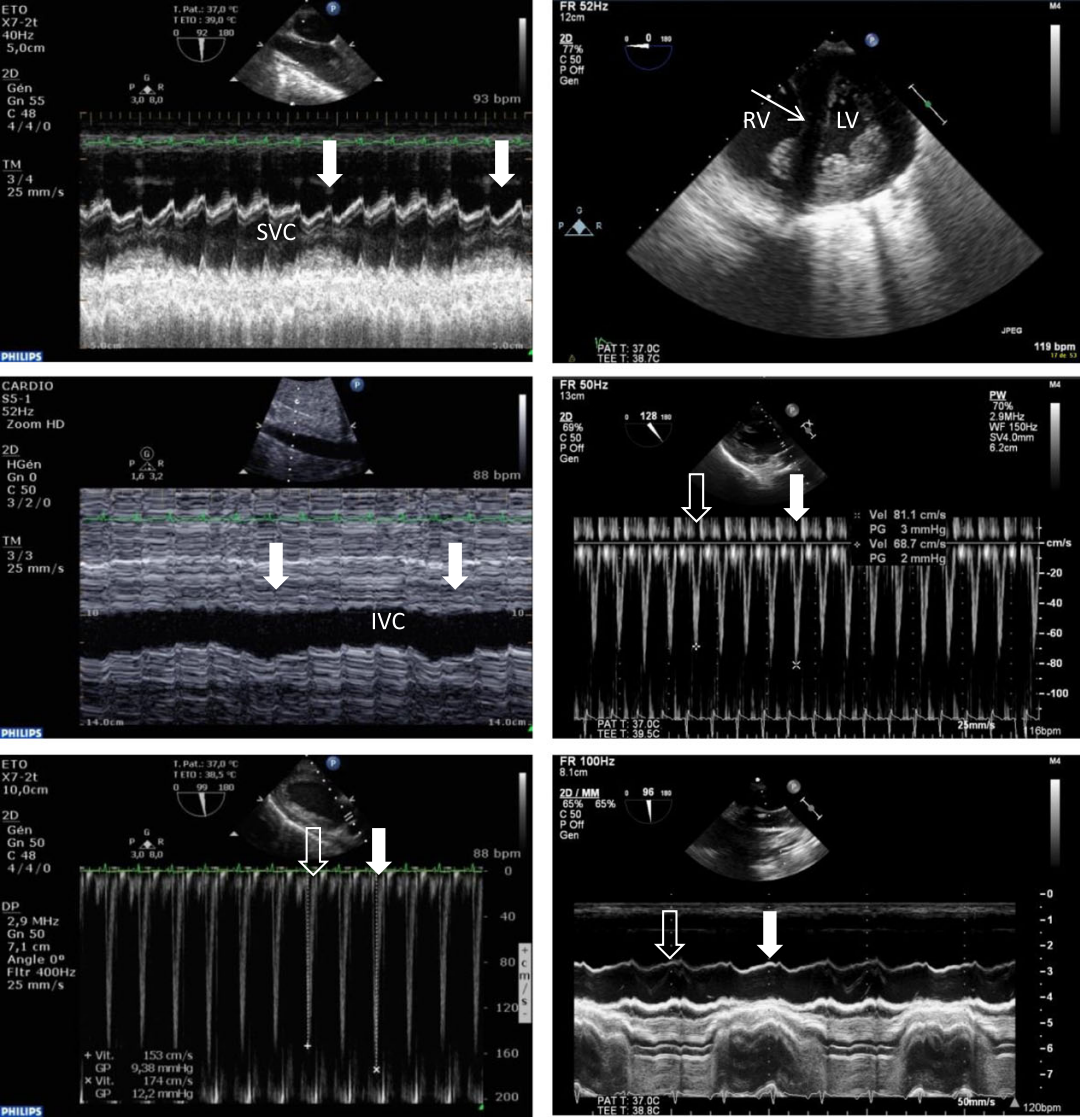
Hemodynamic assessment using transesophageal echocardiography in two patients presenting with shock of distinct origin. In the first patient (left panels), inadequate cardiac output was ascribed to a persisting preload dependence, as reflected by concordant and significant respiratory variations (closed thick white arrow: mechanical insufflation) of the superior vena cava (SVC) diameter (upper left, collapsibility index), of the inferior vena cava (IVC; middle left, distensibility index), and of maximal Doppler velocity recorded in the left ventricular outflow tract (lower left, open thick arrow: expiration phase). In this patient, cardiac output significantly increased after fluid loading. In the second patient who was under protective mechanical ventilation for an acute respiratory distress syndrome (right panels) and exhibited tissue hypoperfusion, a 15% pulse pressure variation suggested fluid responsiveness. Transesophageal echocardiography disclosed an enlarged right ventricle (RV) in conjunction with a paradoxical septal motion consistent with an acute cor pulmonale (upper right, thin arrow) and significant respiratory variations of maximal Doppler velocity recorded in the left ventricular outflow tract (middle right, open thick arrow: expiration phase; closed thick arrow: mechanical insufflation). Nevertheless, the absence of any respiratory variations of the superior vena cava diameter suggested the absence of fluid responsiveness, but rather a systemic venous congestion associated with acute cor pulmonale (lower right). Accordingly, both pulse pressure variation and respiratory variations of maximal Doppler velocity recorded in the left ventricular outflow tract were interpreted as false-positive results. Blood volume expansion was considered potentially inefficient and detrimental, ventilator settings were modified, and inhaled nitric oxide was initiated to further unload the right ventricle. LV left ventricle
